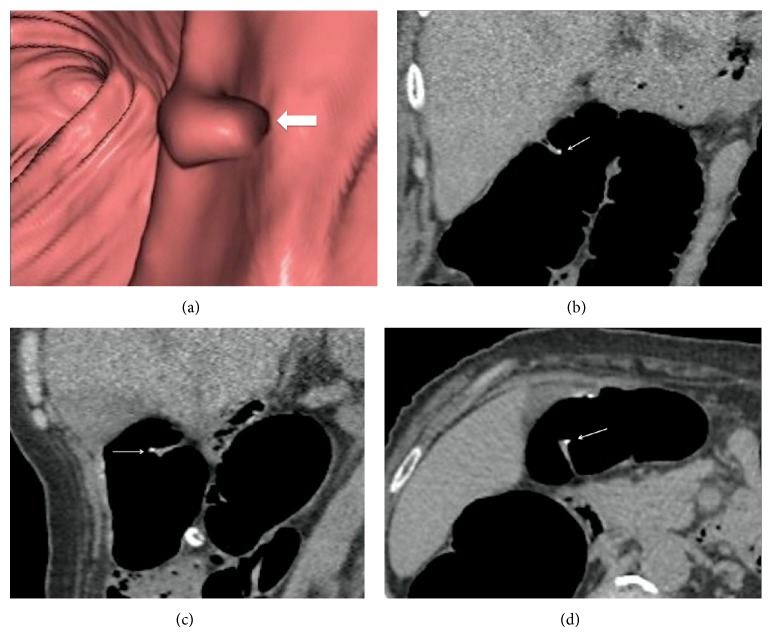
A 62-year-old woman who applied 4-day dietary regimen. 3D endoluminal CT image (a) in supine position shows polypoid structure on haustra of transvers colon (thick arrow) which coronal (b), sagittal (c), and axial (d) CT images confirmed that it was a successfully tagged (score 5) residual solid stool (thin arrow).
